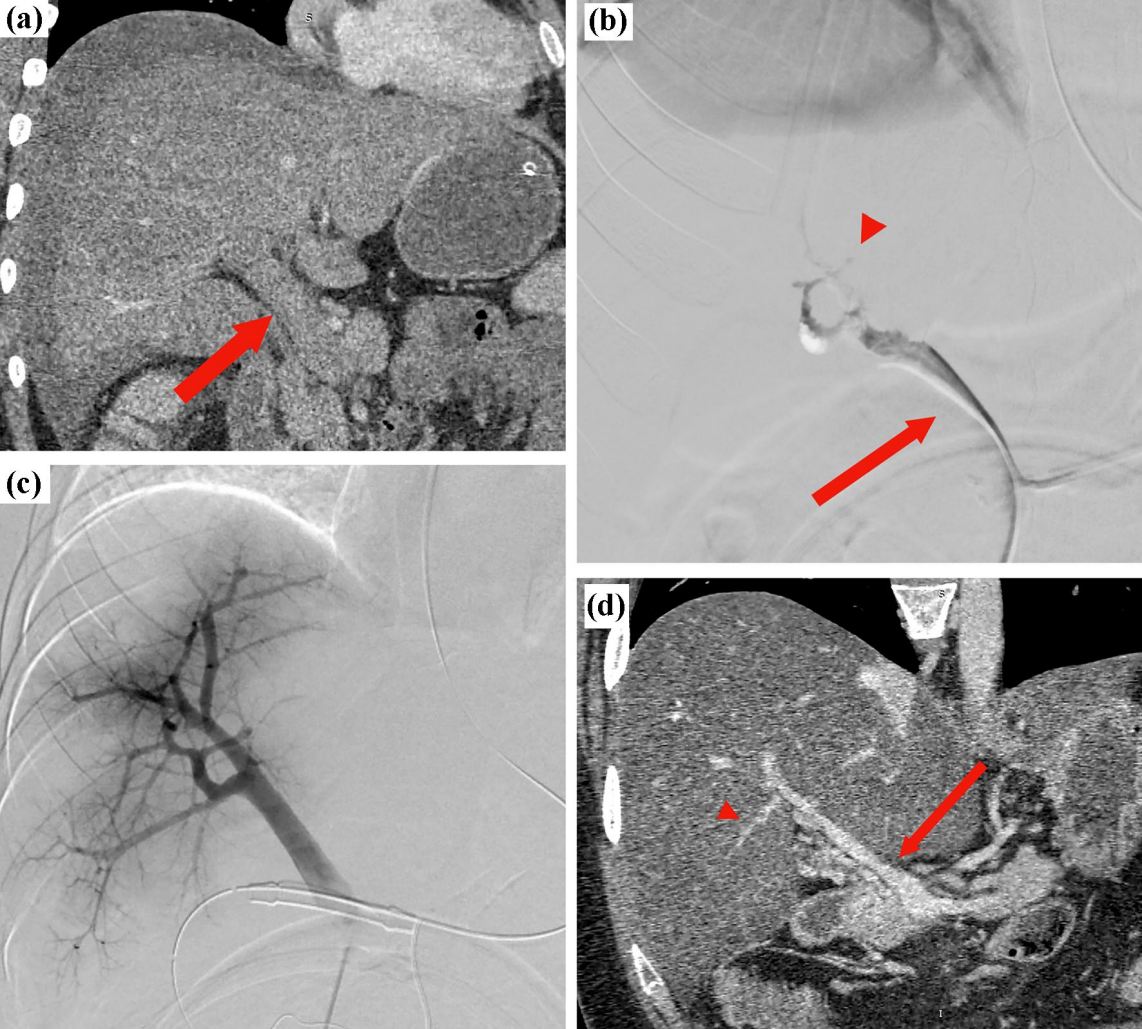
A representative case of the transileocolic approach. A male patient in his early twenties presented with completely occlusive acute portal and mesenteric vein thrombosis with bowel necrosis due to anti-phospholipid antibody syndrome. Transileocolic angioplasty was performed after necrotic bowel resection. a shows completely occluded main trunks of the portal (arrow). b shows the portography via the ileocolic vein. Portography showed completely occluded main trunks of the portal (arrow) and intrahepatic portal veins (arrowhead). c shows the portography after three sessions of temporary endovascular treatments (EVTs) and continuous catheter-directed thrombolysis via the ileocolic vein with open abdomen with vacuum-assisted wound closure in intensive care units. A partial recanalization was performed. d shows the contrast-enhanced computed tomography image obtained 2 years after EVTs. Patency of the main trunk of the portal vein (arrow) and intrahepatic portal veins (arrowhead) was achieved. *CDT* catheter-directed thrombolysis, *CECT* contrast-enhanced computed tomography, *EVT* endovascular treatment
